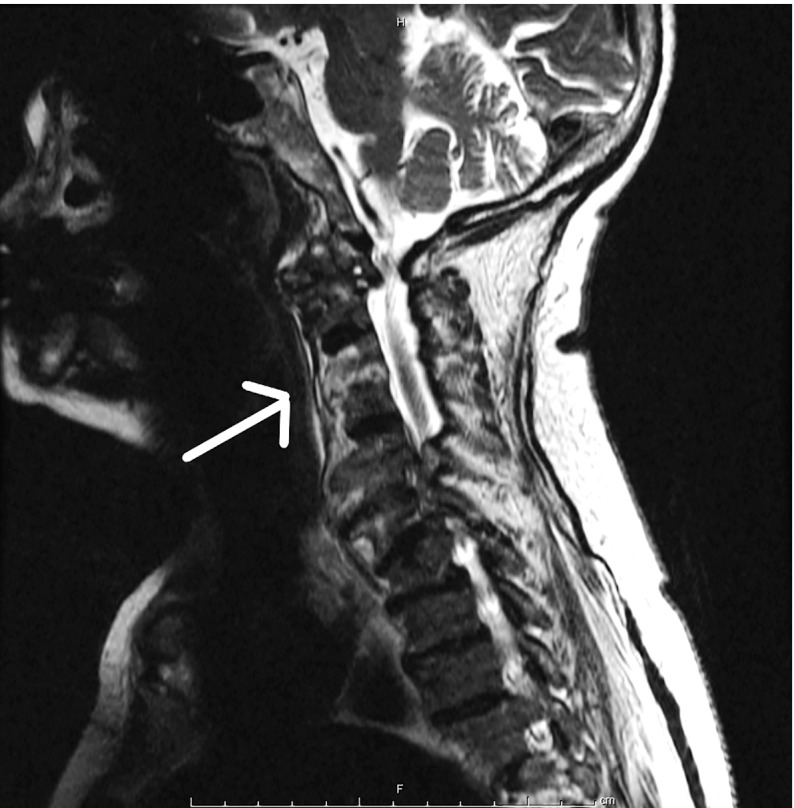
Enhanced T2-weighted magnetic resonance image of the spine of a
